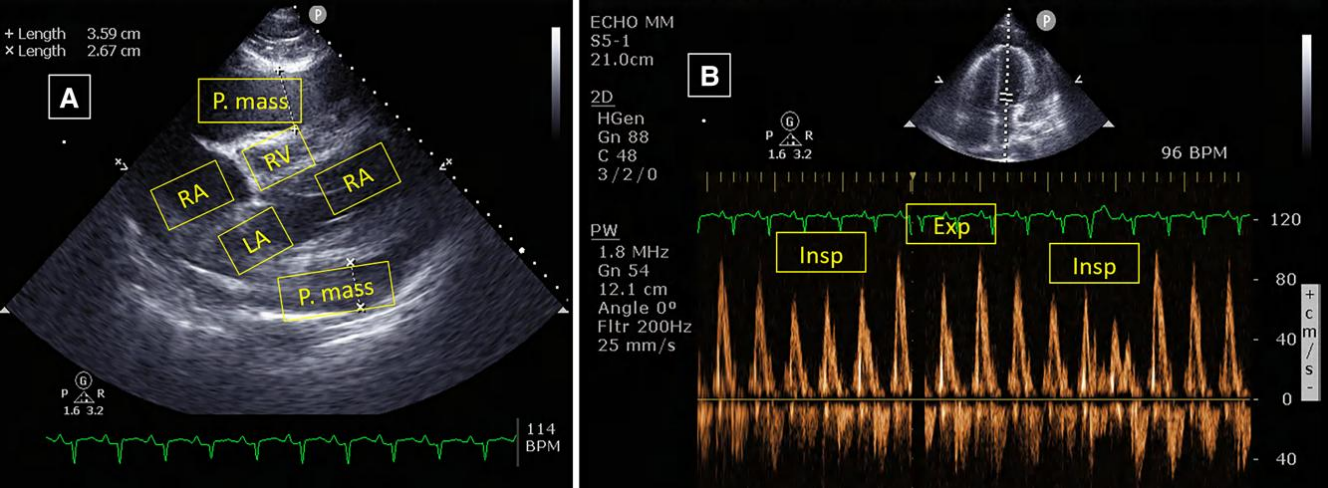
Transthoracic echocardiogram showing large pericardial mass/effusion compressing right ventricle (*A*) with significant respiratory mitral valve flow variation in E-wave velocity with respiration on pulse-wave Doppler (*B*). LV, left ventricle; RA, right atrium; RV, right ventricle.
